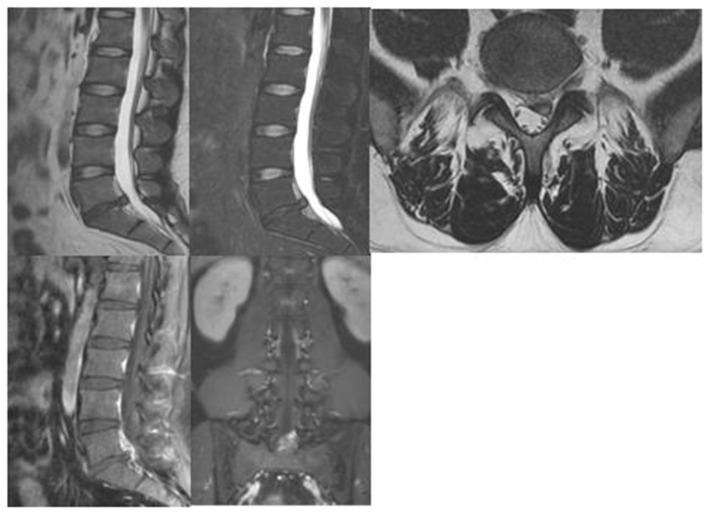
Follow-up lumbar MRI of the same patient 6 months after treatment with the inflammation-preserving protocol, showing a reduction in the L5/S1 disc herniation size. The patient's symptoms of low back pain and left lower limb numbness/pain were significantly improved. The resorption rate reached 61.4%.
